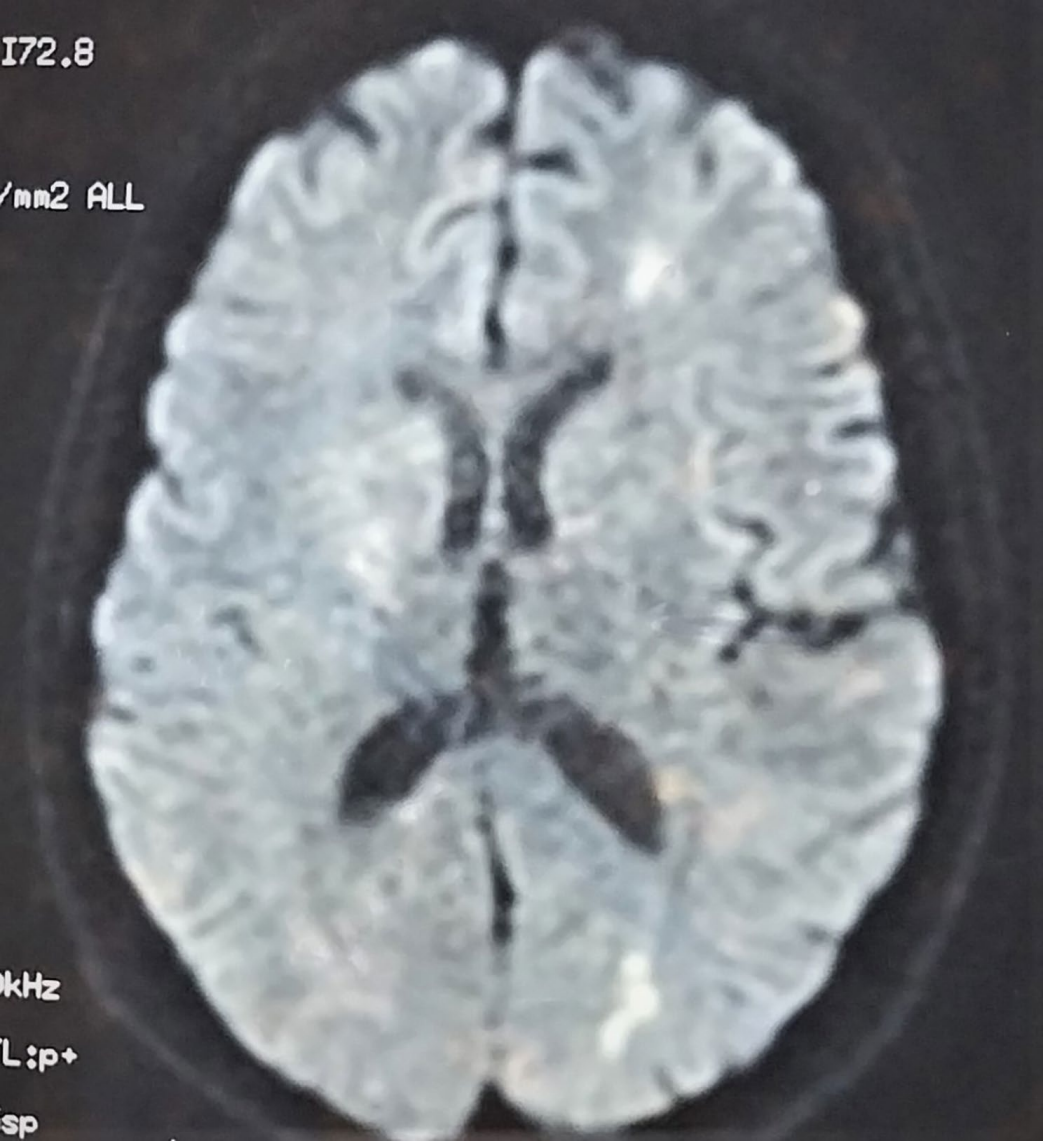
Magnetic resonance imaging (MRI) shows multifocal Hyperintense lesions of the brain (in the left fronto-parietal lobes and the cerebellum) on T2 weighted-images
